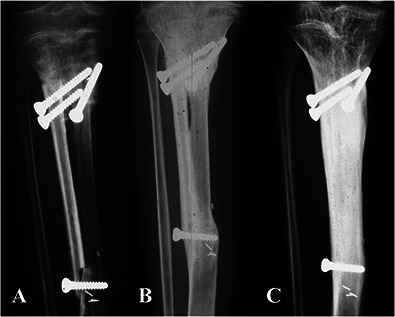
(A) This radiograph is from an 11-year-old girl treated for an osteoblastic osteosarcoma of the tibia with intercalary resection and reconstruction with VFG combined with an allogenic fibular graft and screws-only fixation. Radiographs show (B) progressive hypertrophy of the fibula 2 years after the index surgery and (C) abundant hypertrophy, remodeling, and fusion of the VFG with the cortical strut 5 years after the primary surgery.
